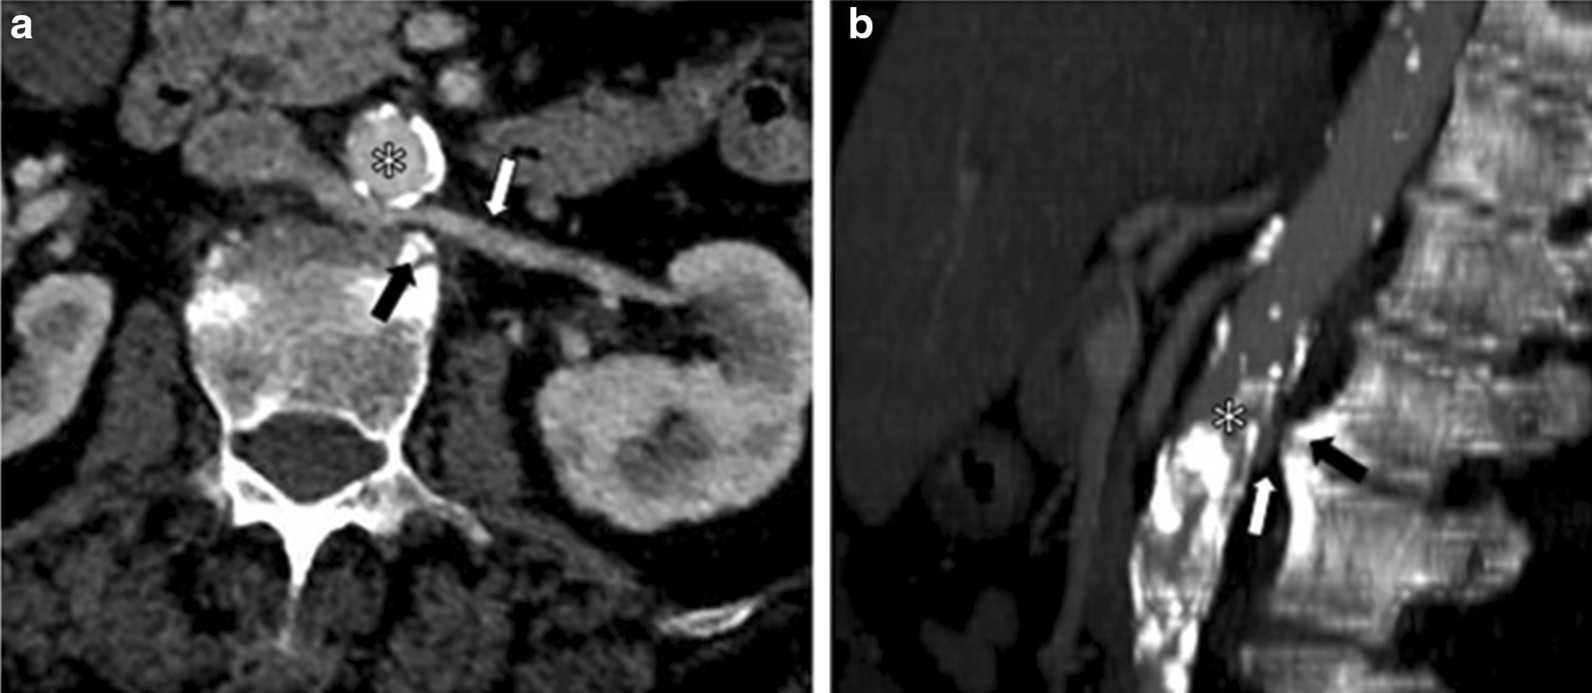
Multidetector CT angiography. a Axial reconstruction and b sagittal reconstruction show the retro-aortic left renal vein (white arrow), which is compressed between the abdominal aorta (*) and osteophyte (black arrow) of the third lumbar vertebral body
